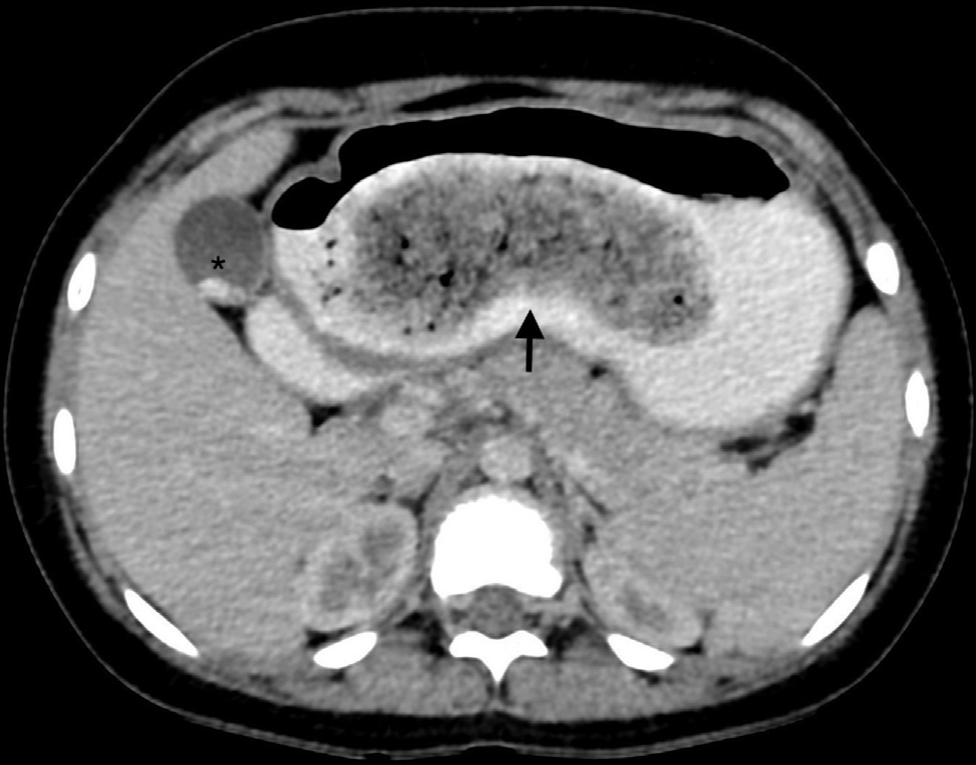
Axial oral and intravenous contrast-enhanced computed tomographic scan of abdomen demonstrating a heterogenous density lesion (black arrow) within the lumen of stomach with “mottled” appearance and clearly outlined by intraluminal oral contrast coincidental gall bladder calculi (asterisk).
